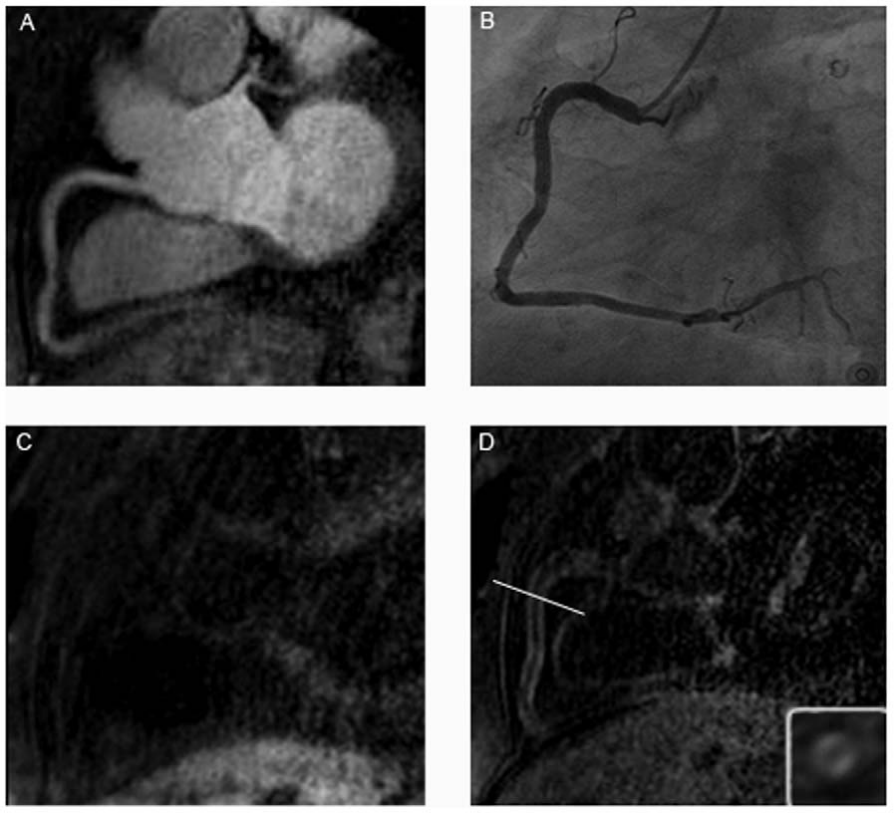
Figure A shows the T2prep image of the RCA in a patient with CAD, while B represents the x-ray angiography and C the IR scan pre and D the IR scan post gadolinium administration in the same patient. At the bottom right of Figure 1D is the enlarged cross sectional view of the appended IR scan.
